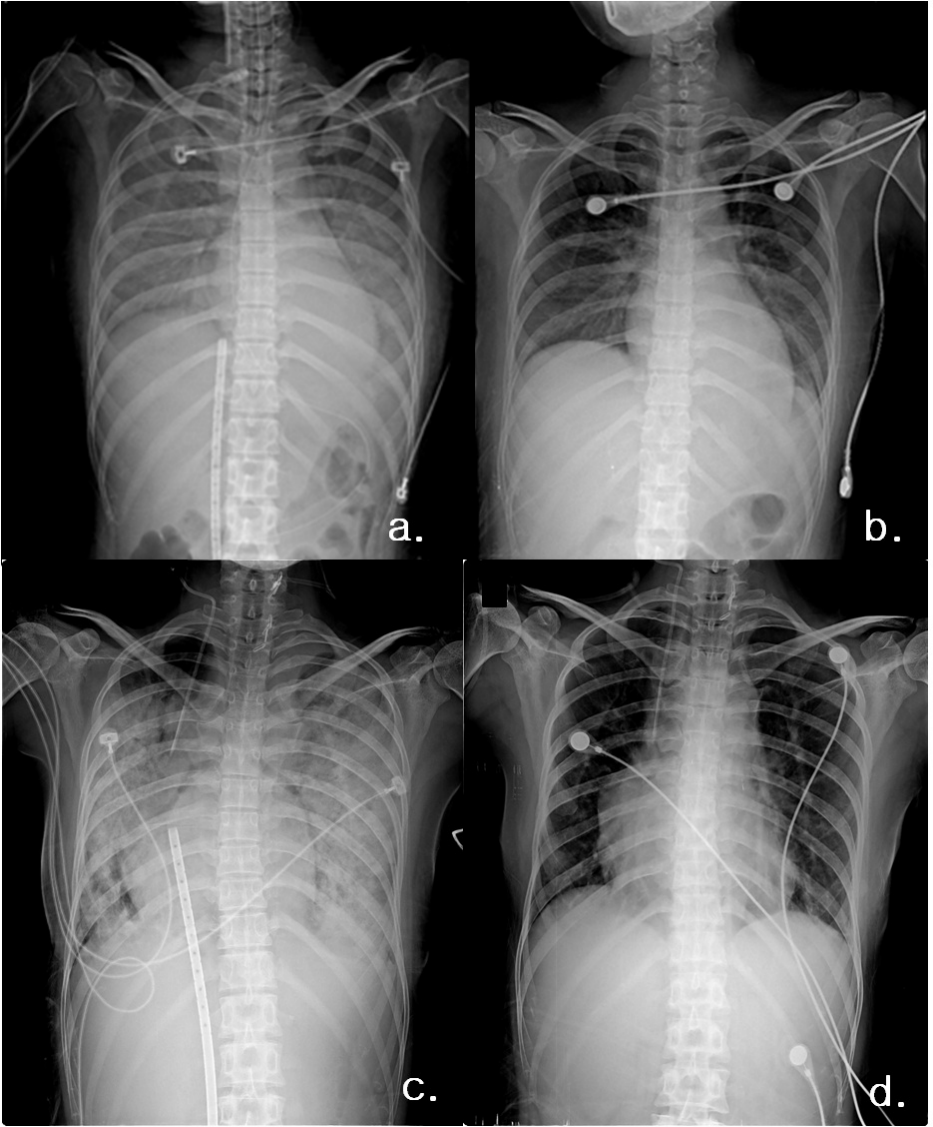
Chest x-ray comparison of both cases. a. Case 1, 3^rd ^day of T-PLS. b. Case 1, 10^th ^day of T-PLS, day of weaning. c. Case 2, 2^nd ^day of T-PLS. d. Case 2, 13^th ^day of T-PLS, one day after weaning.
